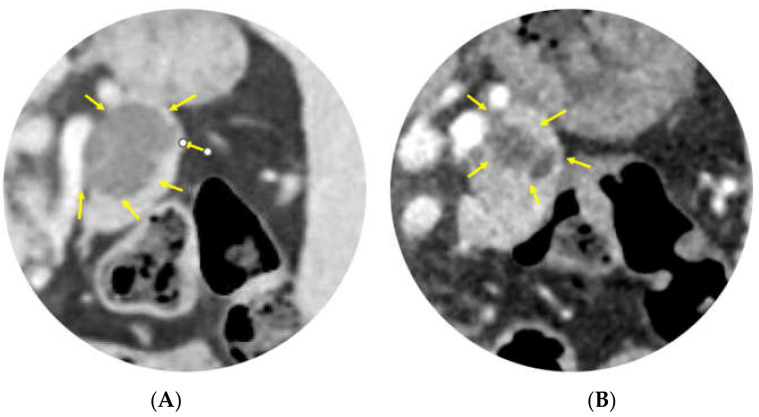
EUS-guided chemoablation with large surface area microparticle paclitaxel (LSAM-PTX) of a branch-duct IPMN. CT-Scan of the abdomen showing a decrease in the lesion size comparing (A) pre-ablation to (B) post-ablation after 2 injections of LSAM-PTX by EUS-FNI.
